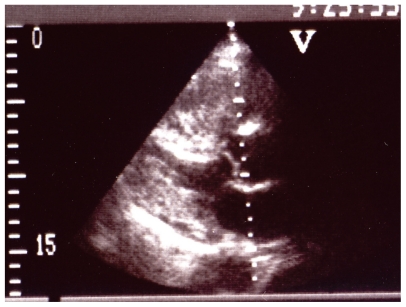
Echocardiogram showing the tumour in the right heart occupying the whole right atrial area.
